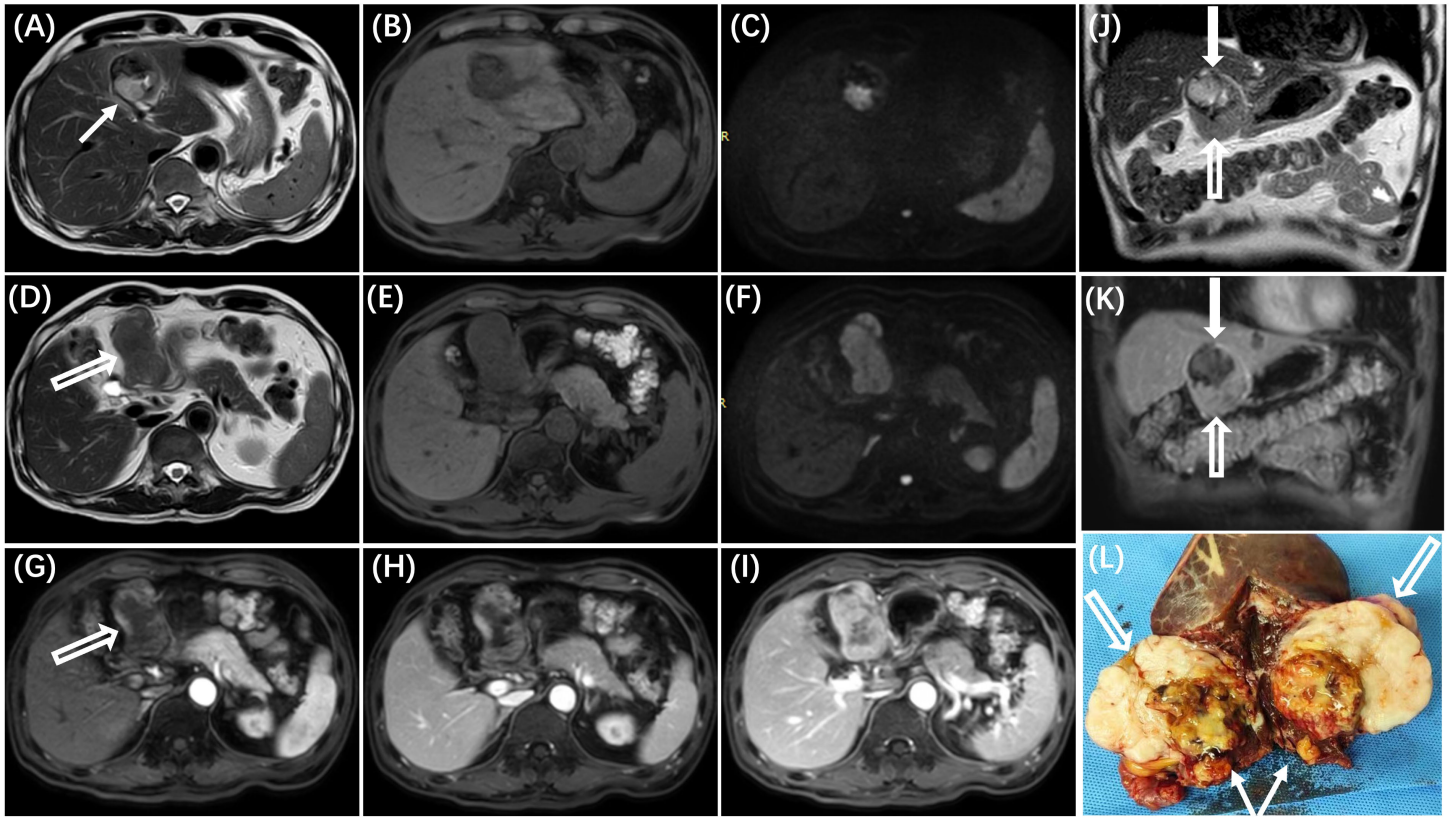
MR imaging reveals two distinct areas within the tumor, each with varying imaging characteristics. The area adjacent to the liver (A–C, white solid arrow in J) demonstrates mixed high and low signals on T_2_WI (A) and T_1_WI (B), corresponding to the undifferentiated region with necrosis and hemorrhage identified in pathological examination (white solid arrow in L). The area distant from the liver (D–F, white hollow arrow in J) exhibits mild hyperintensity on T_2_WI (D) and hypointensity on T_1_WI (E), correlating with the differentiated region observed in pathological examination (white hollow arrow in L). On dynamic contrast-enhanced images, the tumor displays heterogeneous enhancement during the arterial phase (G) and gradual enhancement in the portal venous and delayed phases (H, I), with different degrees of enhancement observed in the two areas (solid arrow and hollow arrow in the K). On diffusion-weighted imaging (DWI), the mass (white arrow) shows hyperintensity (C, F).
